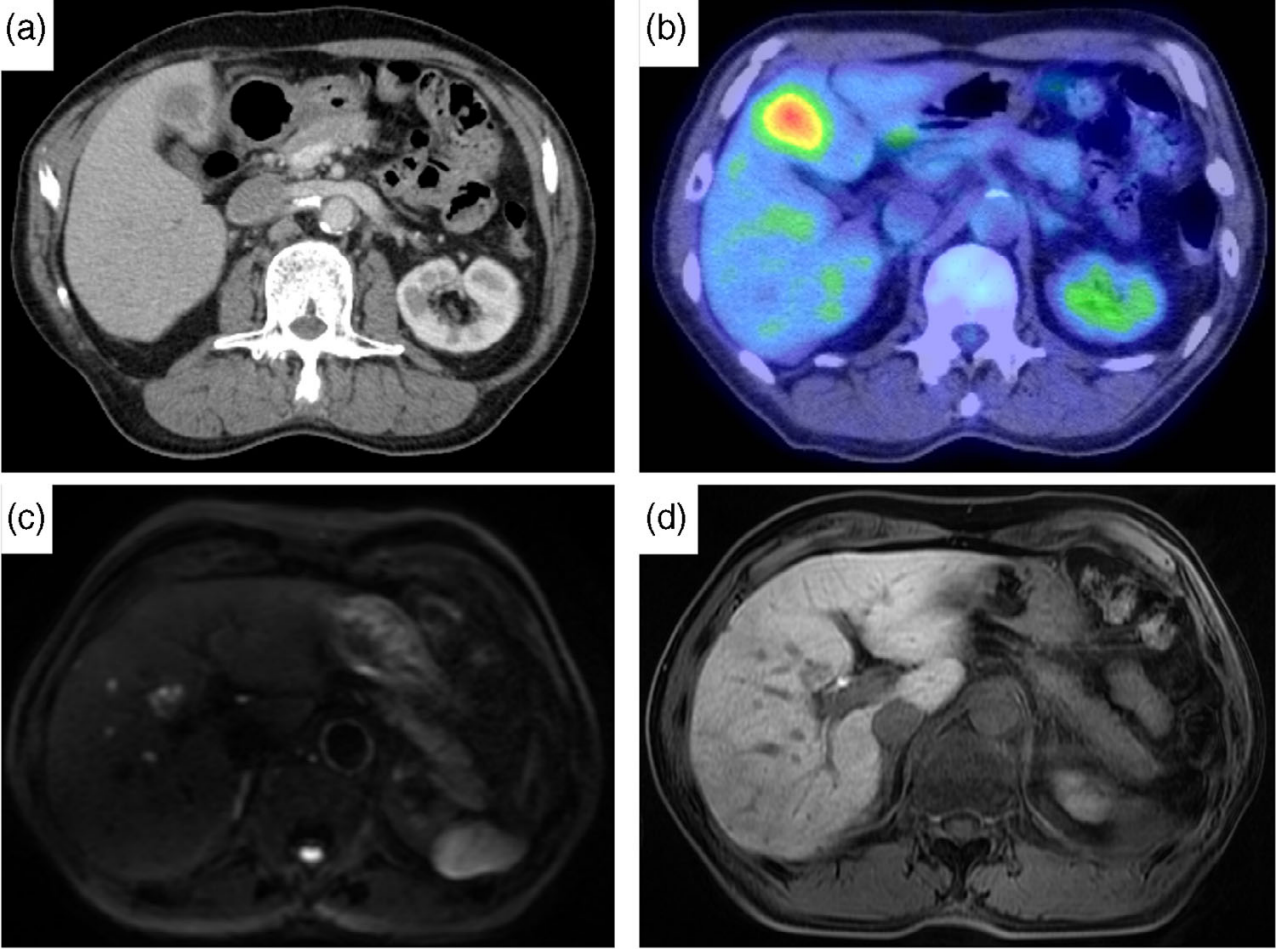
Computed tomography (CT), gadolinium ethoxybenzyl diethylenetriamine pentaacetic acid (Gd‐EOB‐DTPA)‐enhanced magnetic resonance imaging (EOB‐MRI), and 18^F^‐fluorodeoxyglucose positron emission tomography (FDG‐PET)/CT images 18 months after curative endoscopic submucosal dissection. (a) CT revealed a metastatic liver tumor in S4. (b) FDG‐PET/CT showing FDG uptake in the liver. (c, d) EOB‐MRI revealed metastatic liver tumors in S4, S5, and S8.
